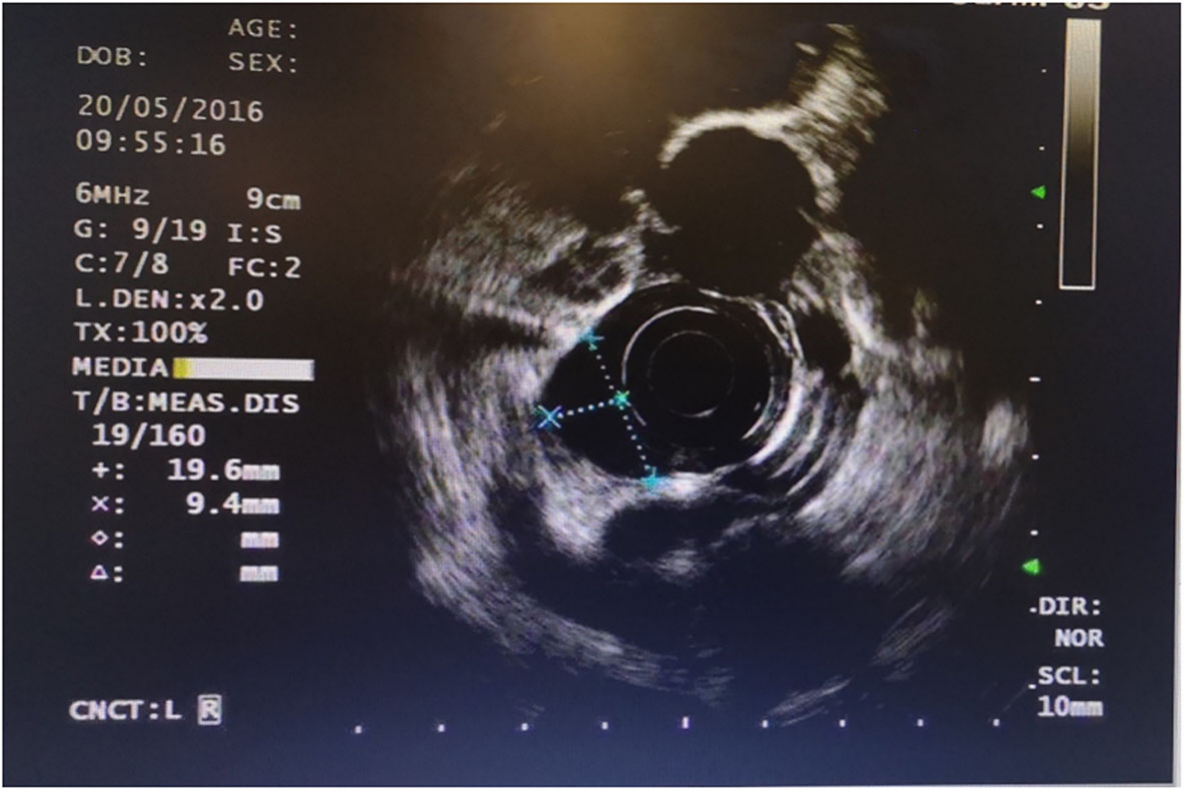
Endoscopic ultrasound showed the anechoic mass arising from muscularis propria, with the size of about 3.0*2.0 cm
